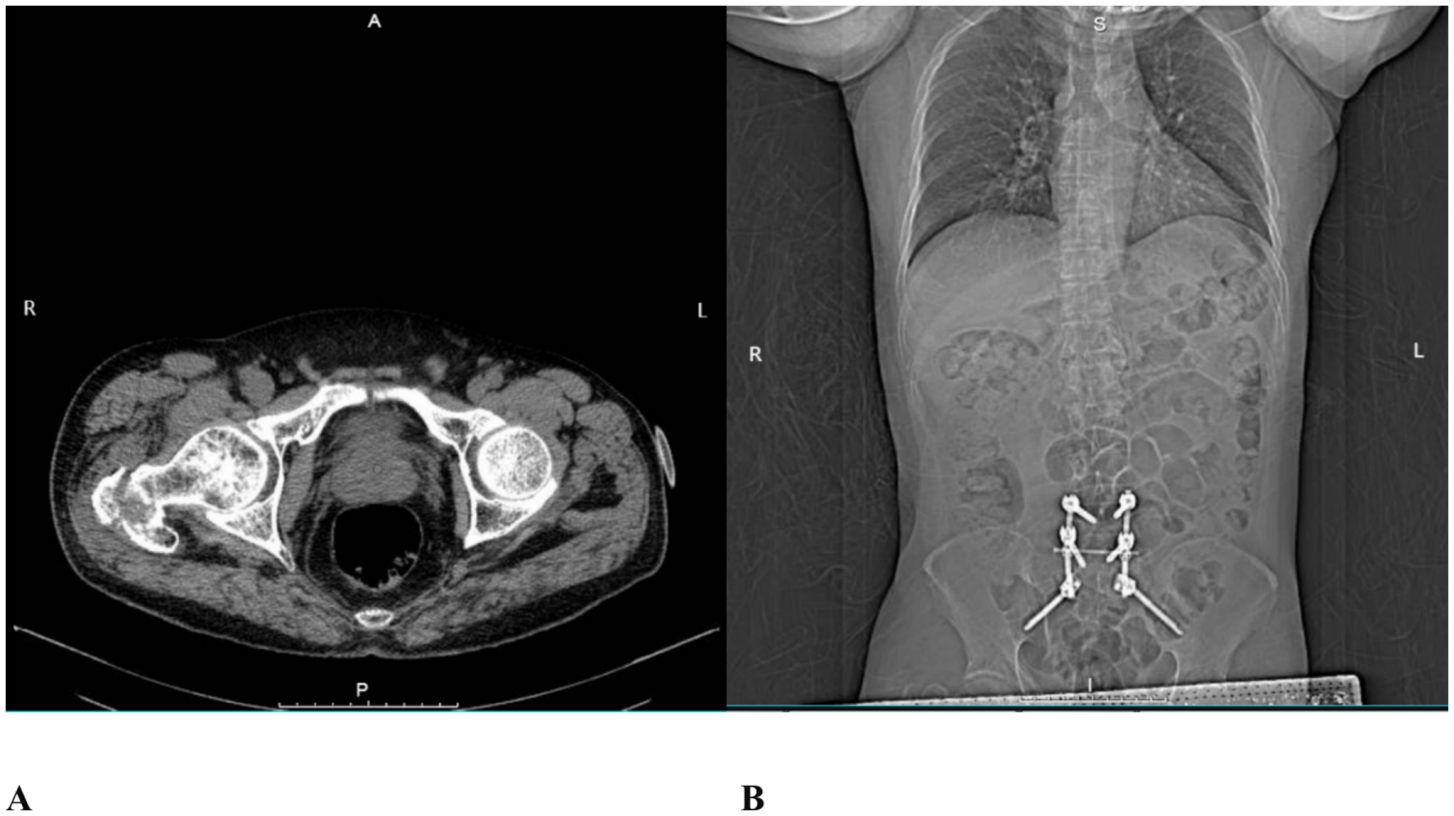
CT images. (A) Basal fracture of the right femoral neck; (B) Postoperative imaging following prior posterior L5/S1 lumbar interbody fusion.
